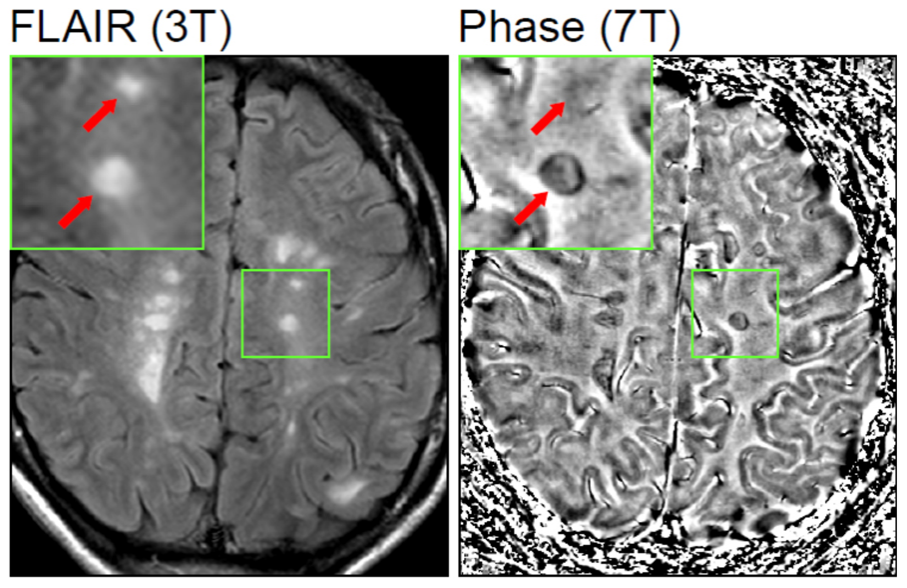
FLAIR (3T) and GRE phase (7T) images of a patient with active relapsing-remitting MS. FLAIR images show numerous white matter MS lesions of which 2 are magnified (inset, red arrows). Phase imaging at 7T phase/GRE reveals a hypointense ring corresponding with one lesion on FLAIR. The other lesion is not visible on 7T GRE (inset, arrows).
